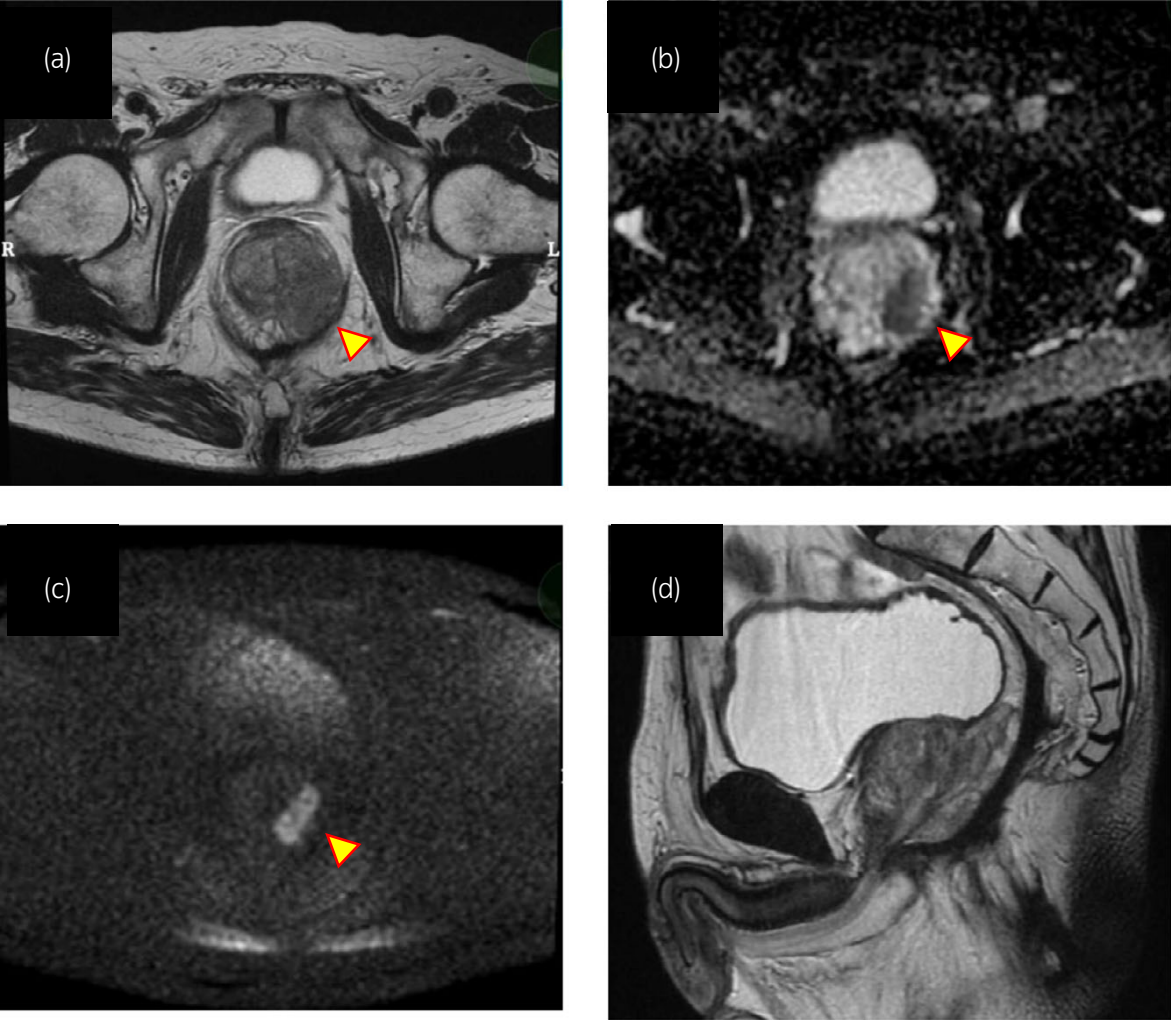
Imaging findings of the prostate. Multiparametric MRI demonstrated a well‐defined lesion in T2 (a), diffusion (b), and apparent diffusion coefficient (c) axial sections in the left peripheral zone, with overall features of a PIRADS 5 lesion. T2 sagittal section of prostate (d).
